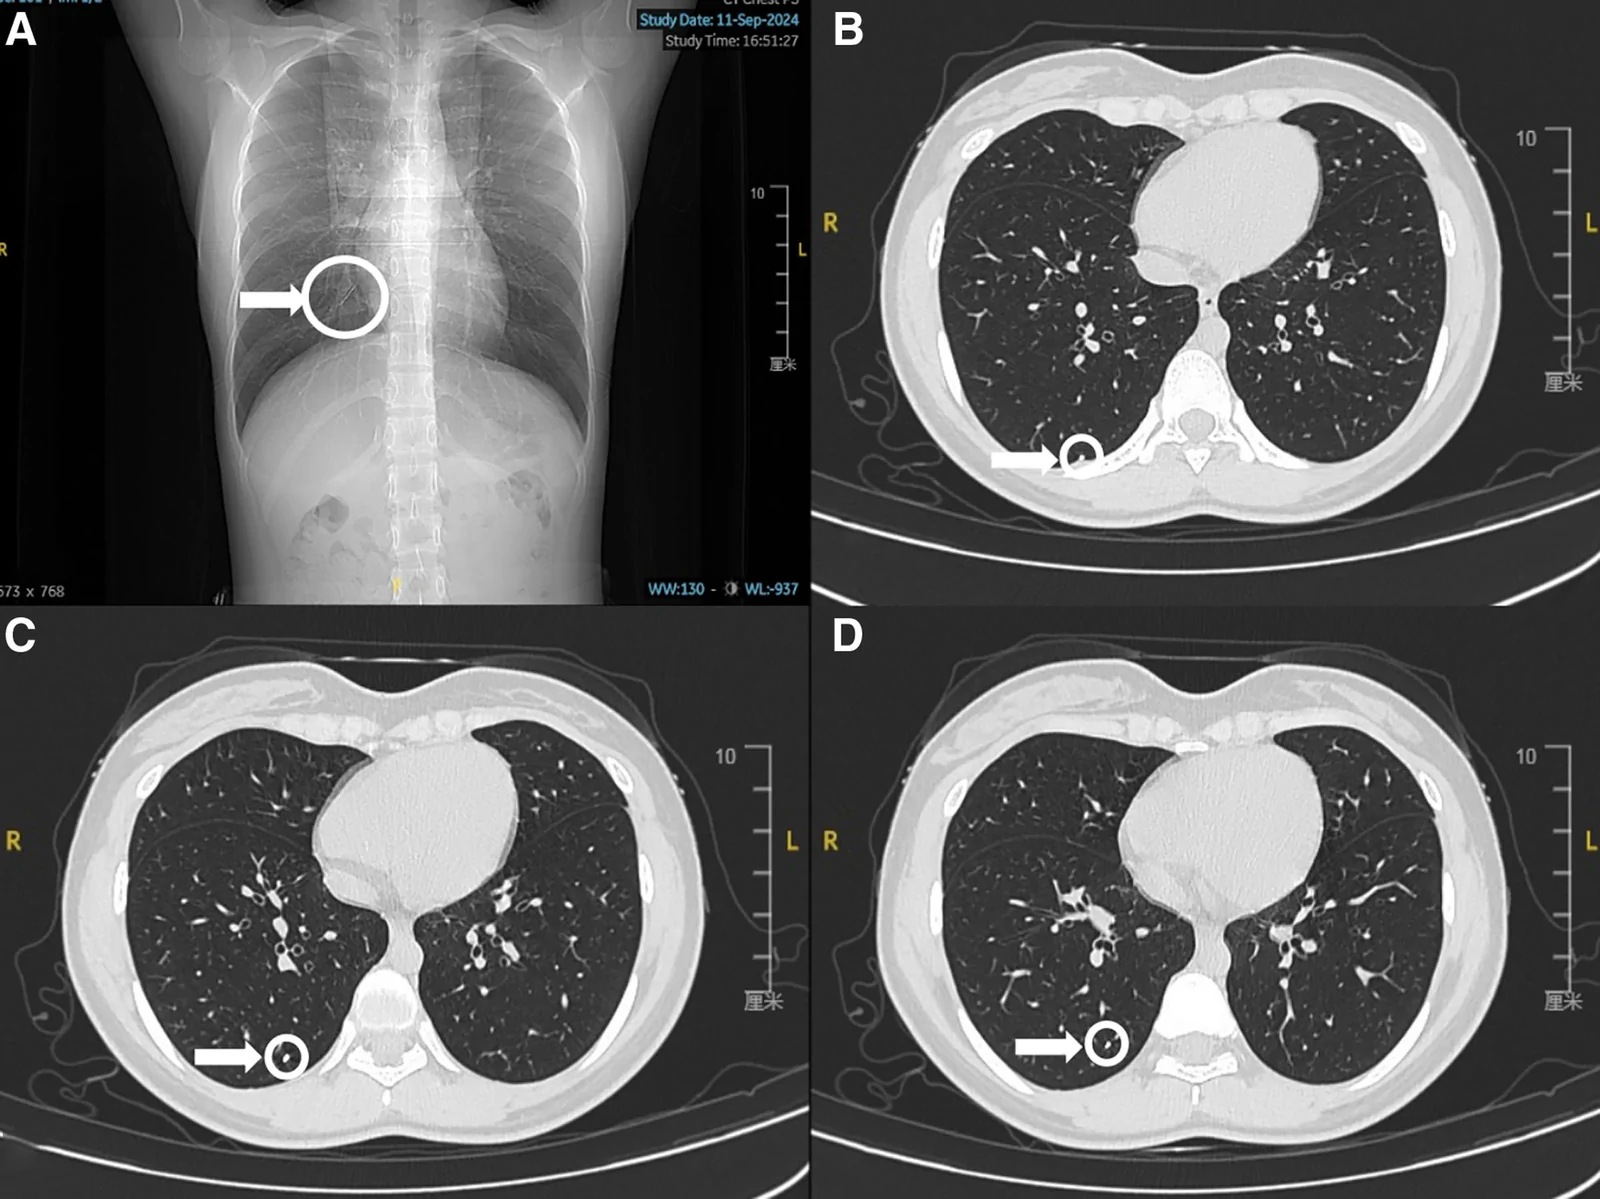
Preoperative radiological assessment. (A) Chest X-ray revealing a high-density foreign body in the right lower lung field (indicated by a white circle). (B–D) Axial thin-slice CT images demonstrating the trajectory of the sewing needle penetrating from the pulmonary surface to the deeper parenchymal tissue of the right lower lobe (white circles denote the sectional appearances of the sewing needle). CT = computed tomography.
